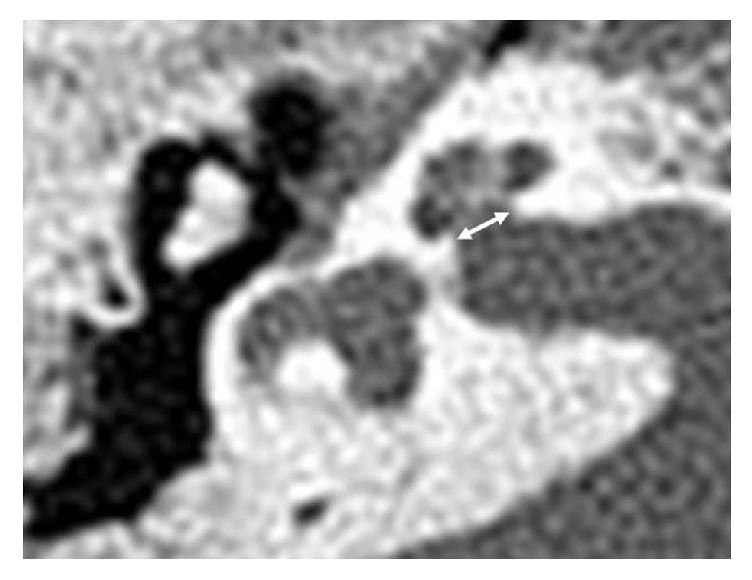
Axial slices of temporal bone computed tomography for the measurement of bony cochlear nerve canal (BCNC) in normal bony cochlear nerve canal. The width was measured by the distance between the inner margins of bony walls at midportion, at the fundus level of cochlear nerve in internal auditory canal.
